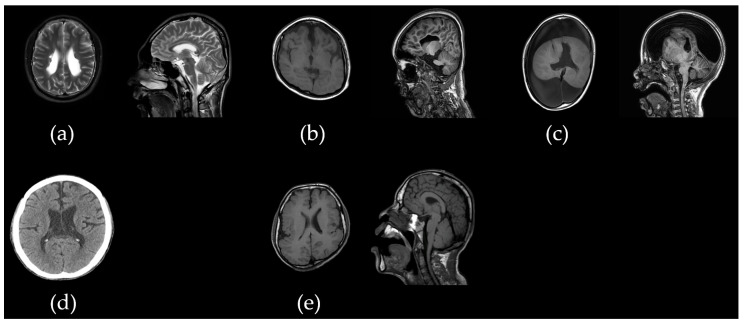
Brain magnetic resonance imaging features of atypical cerebral palsy. (a) Patient acp02s showed periventricular irregularities with associated hyperintensities on T2WI. (b) Patient acp03k showed frontotemporal atrophy with periventricular ischemic or demyelinating changes. (c) Patient acp05y showed hydranencephaly and lobal holoprosencephaly with open-lip schizencephaly with associated callosal agenesis and cortical malformation. (d) Patient acp08p showed widening of the side ventricles. (e) Patient acp09k showed schizencephaly with cortical dysplasia.
